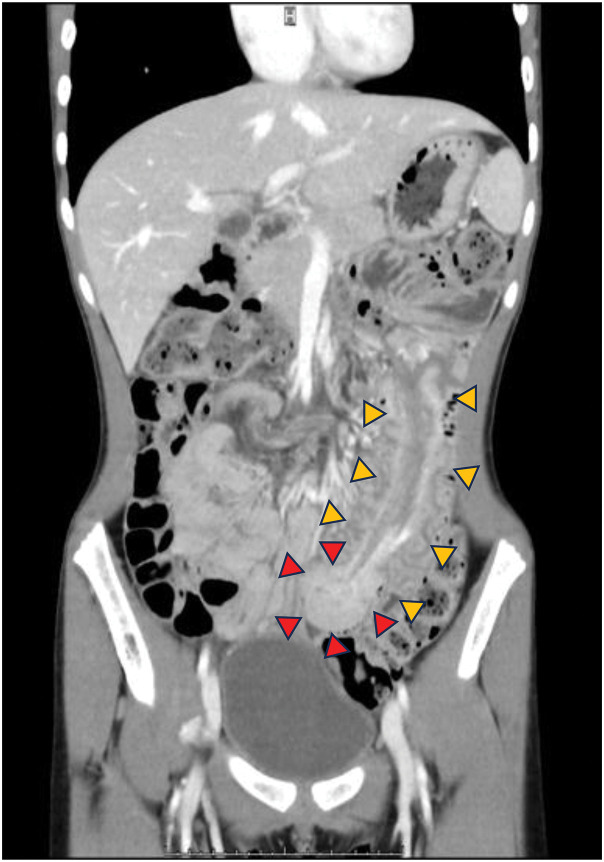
CT image of intestinal intussusception caused by a polyp. A CT scan showed an intussusception of the small bowel (yellow arrowheads) with a mass (red arrowheads) in the advanced region.
